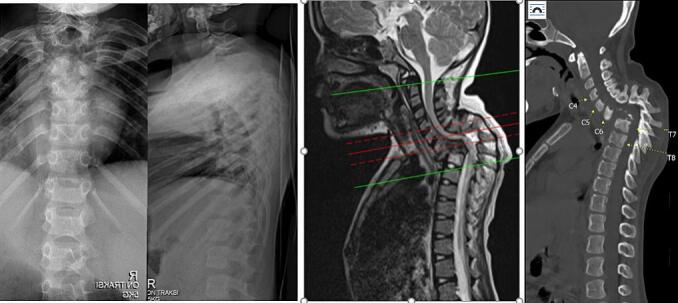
Radiologic examination on the patient, (A) thoracolumbal view of plain radiographic, (b) Sagittal view of non-contrast cervicothoracal MRI, (c) Sagittal view of cervicothoracal CT Scan.
